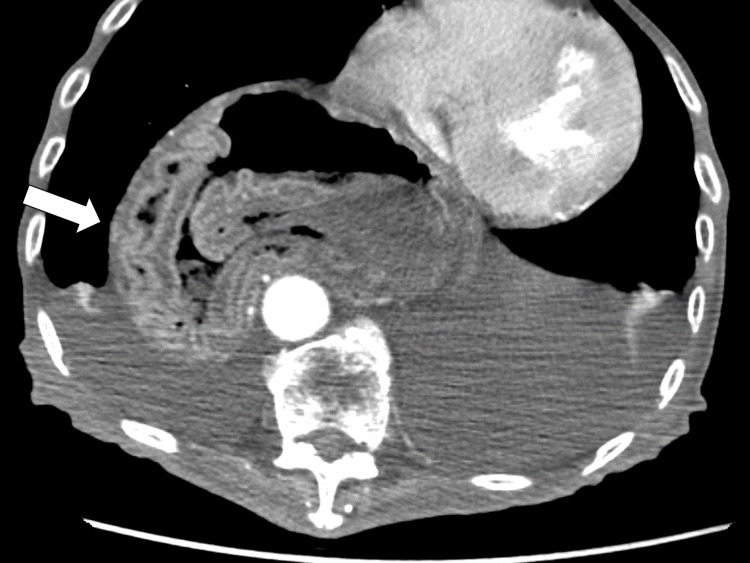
Contrast CT scan on the 17th day The CT revealed gastric wall thickening. CT, computed tomography
